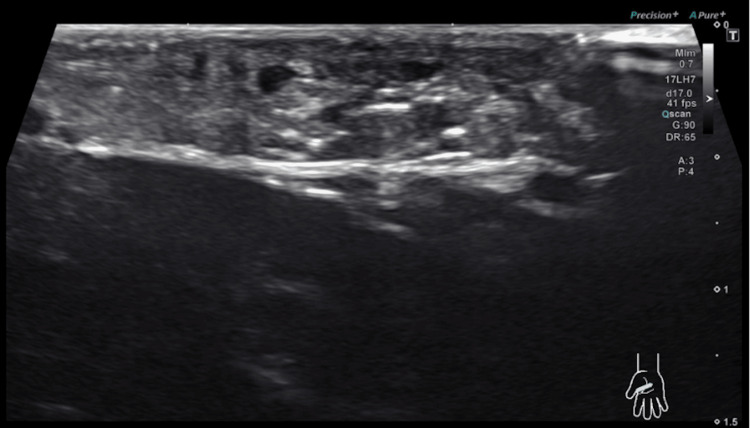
Ultrasound image in the oblique section of the palmar abnormality. This image demonstrates echogenicity and distortion of the normal fascial planes, further supporting a fibrotic contracture of the first web space.
